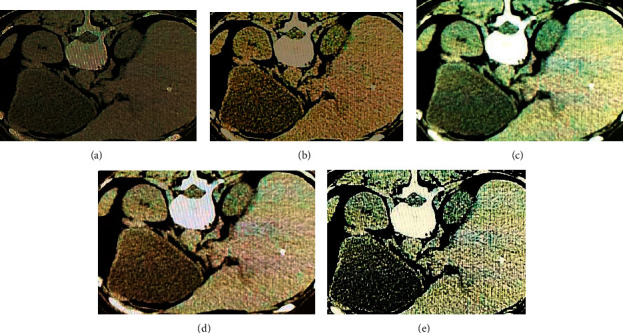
Results of reconstructed CT images by four algorithms. (a) The original image; (b, c, d, and e) the reconstructed images processed by the TV model, FBP algorithm, WTV model, and L0-ART algorithm, respectively.
